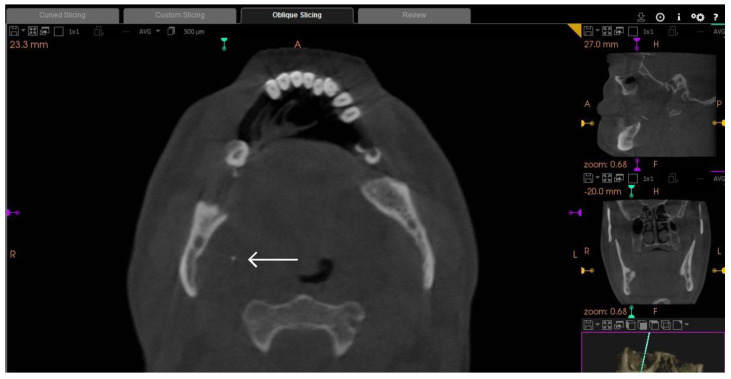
Axial section of CBCT taken immediately after needle fracture showing the anterior portion of the needle (arrow). The needle was directed obliquely vertical to the point of insertion and medial to the medial pterygoid muscle. Fracture of local anesthetic needles during injection has been described, particularly during the inferior alveolar nerve block, which is considered the most common local anesthetic procedure to be used intraorally, and to be complicated by needle fracture [1]. The incidence is reported to be 1 in 14 million inferior alveolar nerve blocks [2]. Upon the occurrence of this unfortunate event, the surgeon is faced by a number of options: to leave in situ or, more favorably, to remove as soon as possible to avoid the anticipated complications of pain, trismus, infection, and damage to important structures of blood vessels and nerves [3]. Needles broken during inferior alveolar nerve block may migrate in the pterygomandibular space where the mandibular nerve and inferior alveolar blood vessels are located, which presents a surgical challenge because of the interplay of complicated access, the close anatomic relationship to important structures, and the small diameter of the anesthetic needle fragment This report describes a case of a local anesthetic needle that was broken during injection procedure. It also describes diagnostic methods utilized and the surgical method that was followed to retrieve the broken needle. A 50-year-old, medically fit non-smoking male presented for the extraction of his lower-right third molar which had been affected by severe caries. Upon initiation of the inferior alveolar nerve block anesthesia using a disposable 23 mm 30-gauge dental needle, the patient developed a gag reflex and suddenly moved his head. The surgeon attempted to remove the needle; however, the needle fractured and the broken needle was out of sight. The surgeon estimated that the broken needle disappeared completely in the pterygomandibular space medial to the ramus. A decision was made to take a CBCT scan (Cone beam computed tomography) immediately to visualize the broken segment. (Figure 1).
